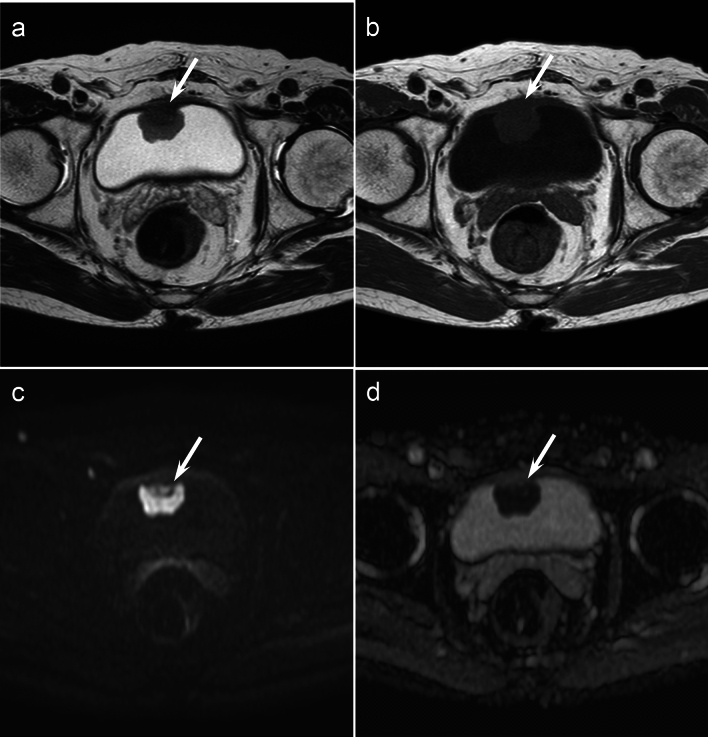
A 77-year-old man with bladder SCNEC. Axial T2-weighted image a and Axial T1-weighted image b indicate a pedunculated bladder mass (arrow) with a smooth lobulated margin and without extension into perivesical fat. c Axial diffusion-weighted image shows a mass that is markedly hyperintense (arrow). d Axial ADC map shows markedly restricted diffusion, with decreased ADC values (0.496 × 10^−3^ mm^2^/s) (arrow)
